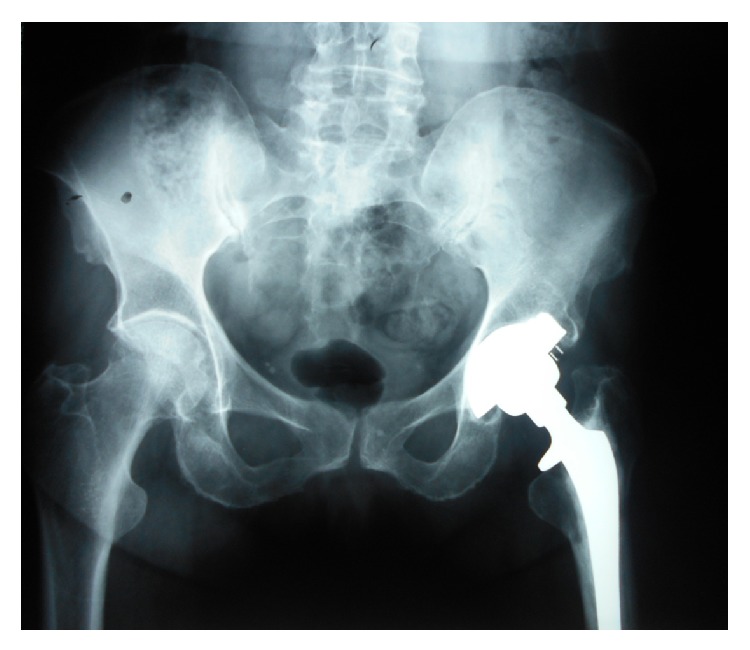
Anterior-posterior hip X-rays in patient number 4 after 5 years on Coumadin. The right hip is unchanged, Ficat stage II. The left hip, Ficat stage III at preanticoagulation study entry, was replaced before study entry.
